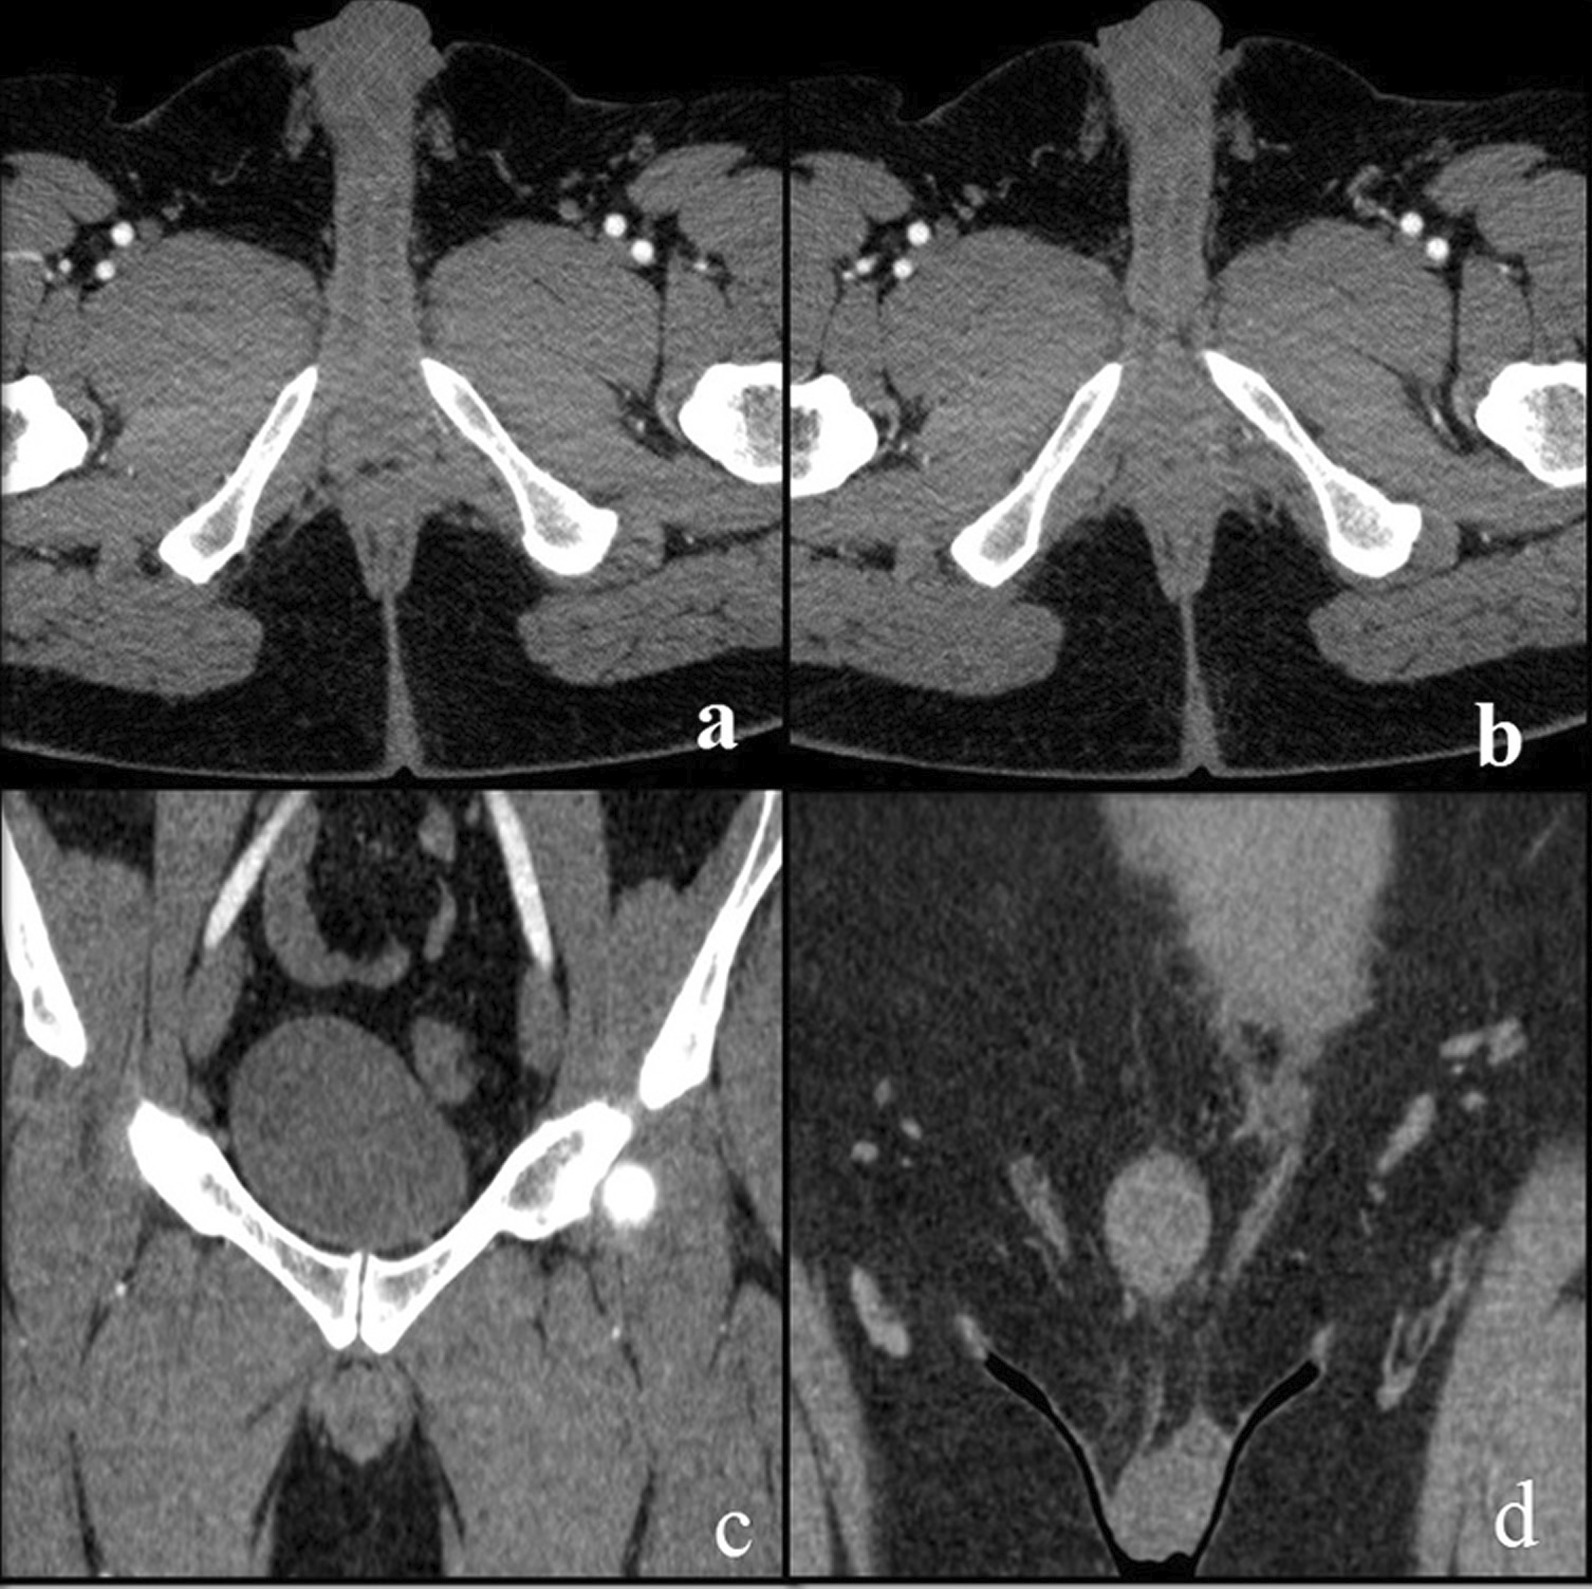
a–d Postoperative pelvic CT angiography images a 42-years-old male. Penil vascularity was prominently diminished and vascular structures were not observed
